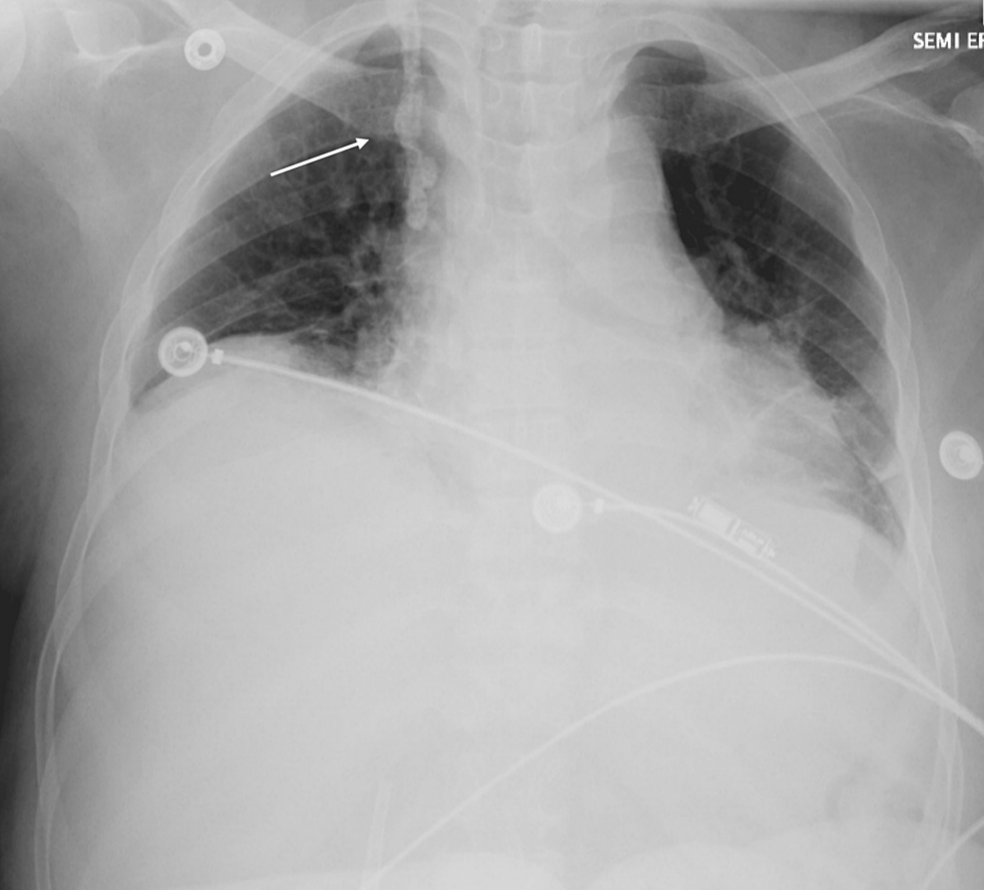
Chest x-ray demonstrating calcific density projecting over the right lower neck
